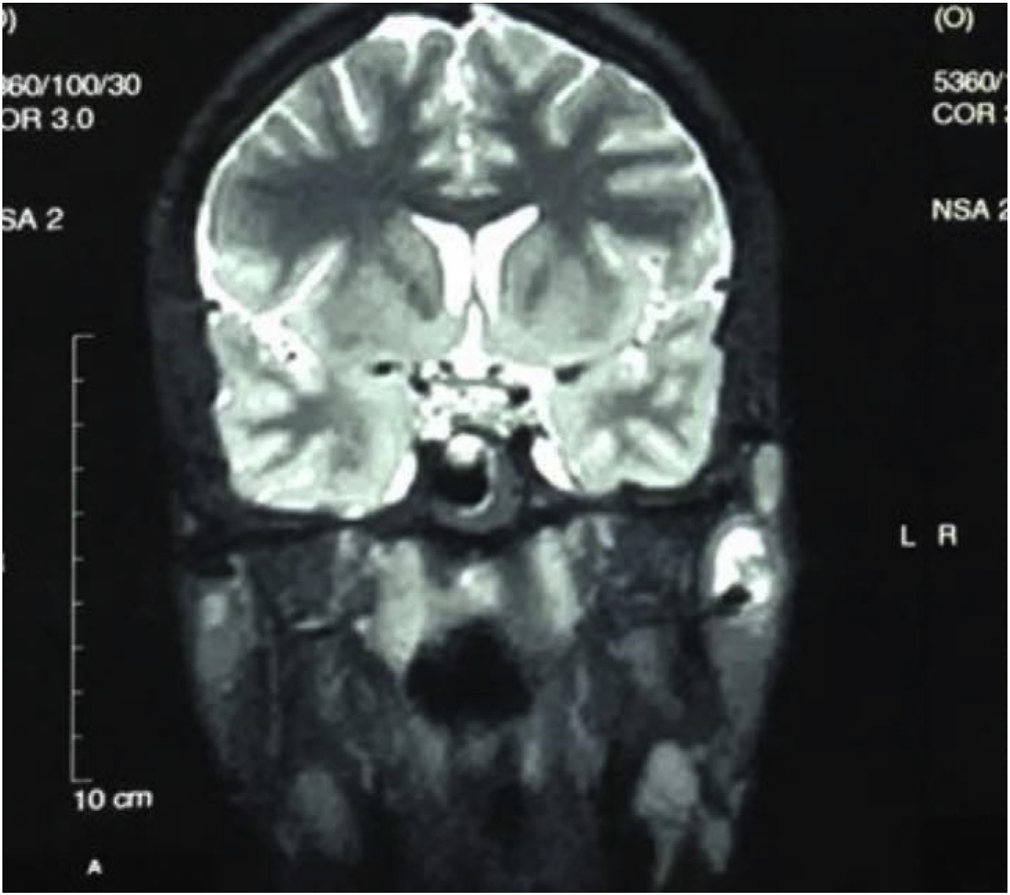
MRI T2 coronal section: visualisation of T2-hyperintense arterial blood flow (arrow) within a T2-hypointense parotid mass.
